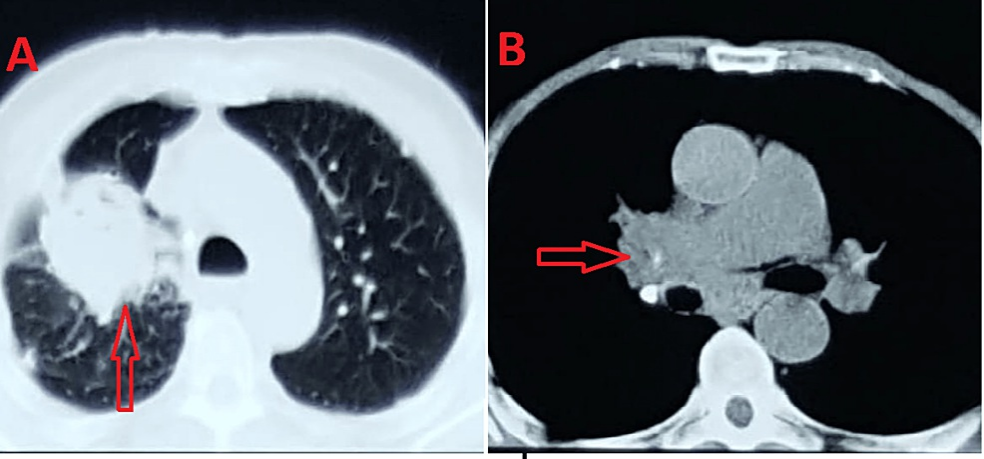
CT scan with red arrows pointing to lung mass. A: axial section with parenchymal window. B: axial section with mediastinal window.
